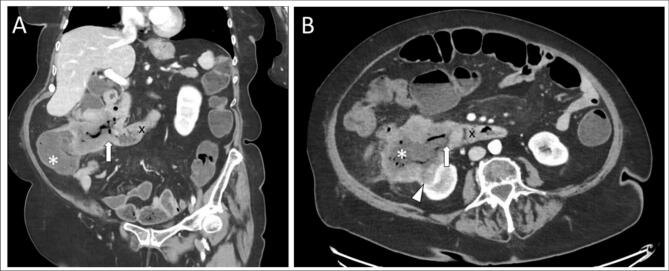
Initial coronal (A) and axial (B) computed tomography (CT) scan of the abdomen, with a tumor arising from the ascending colon (*), with local invasion of the right kidney (white arrowhead) and a malignant duodenocolic fistula (thick white arrow), connecting the ascending colon with the duodenal tract (x).
